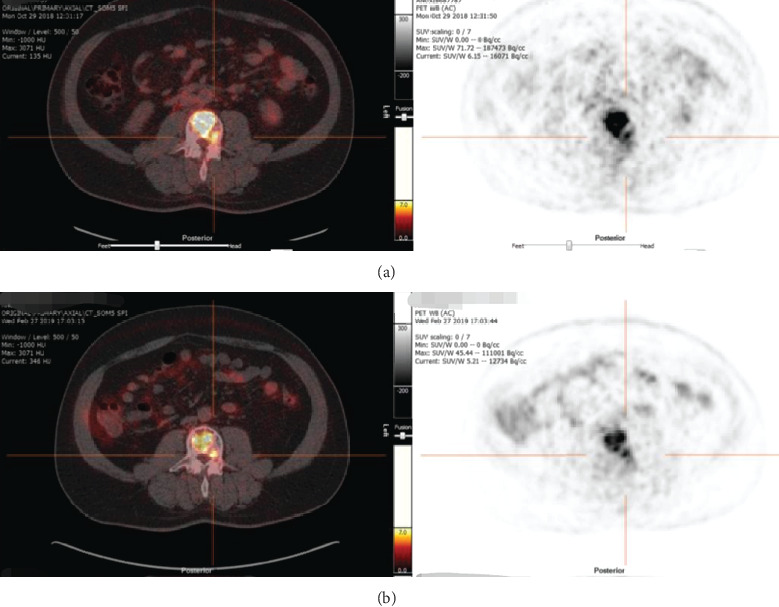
^18^F-FDG PET/CT scan showing the difference in SUV maximum for lesion located in C6 at the moment of diagnosis (a) vs SUV maximum for same location after 3-cycle of Lenvatinib oral treatment (b). The decrease was quantified in 39.5%.
